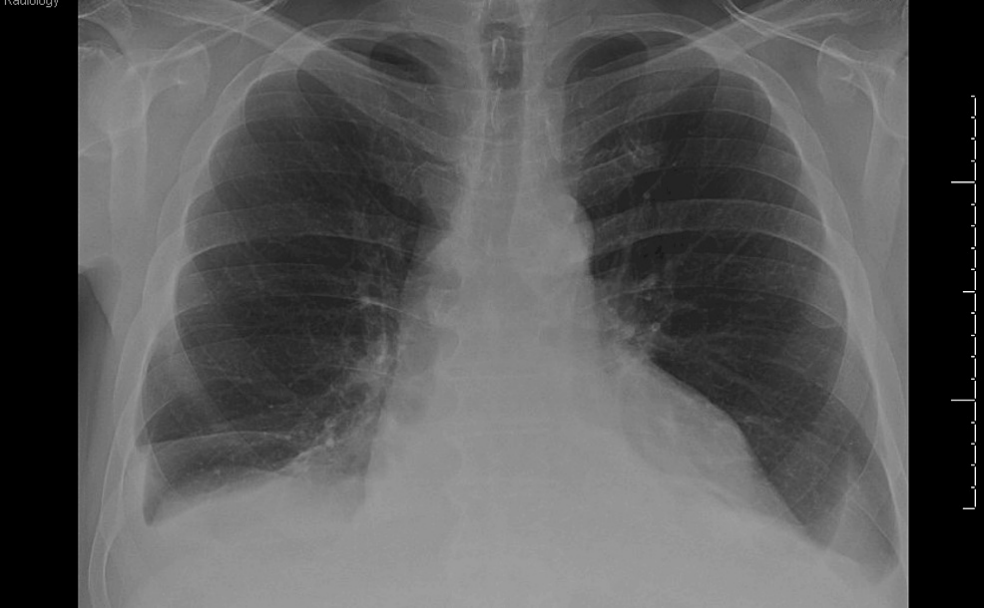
Chest x-ray depicting a small bilateral pleural effusion.
